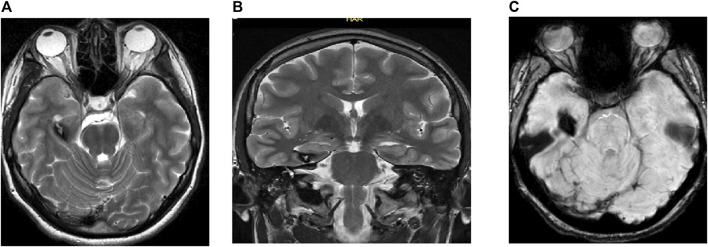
MRI of the brain of a 17-year-old man showing (A) axial T2 weighted image (WI), (B) coronal T2 WI, and (C) gradient echo (GRE) T2*/SWI: Figure show RT hippocampal popcorn lesion with peripheral signal loss due to hemosiderin deposition and prominent blooming in the GRE T2*/SWI image, characteristic of hippocampal cavernoma.
